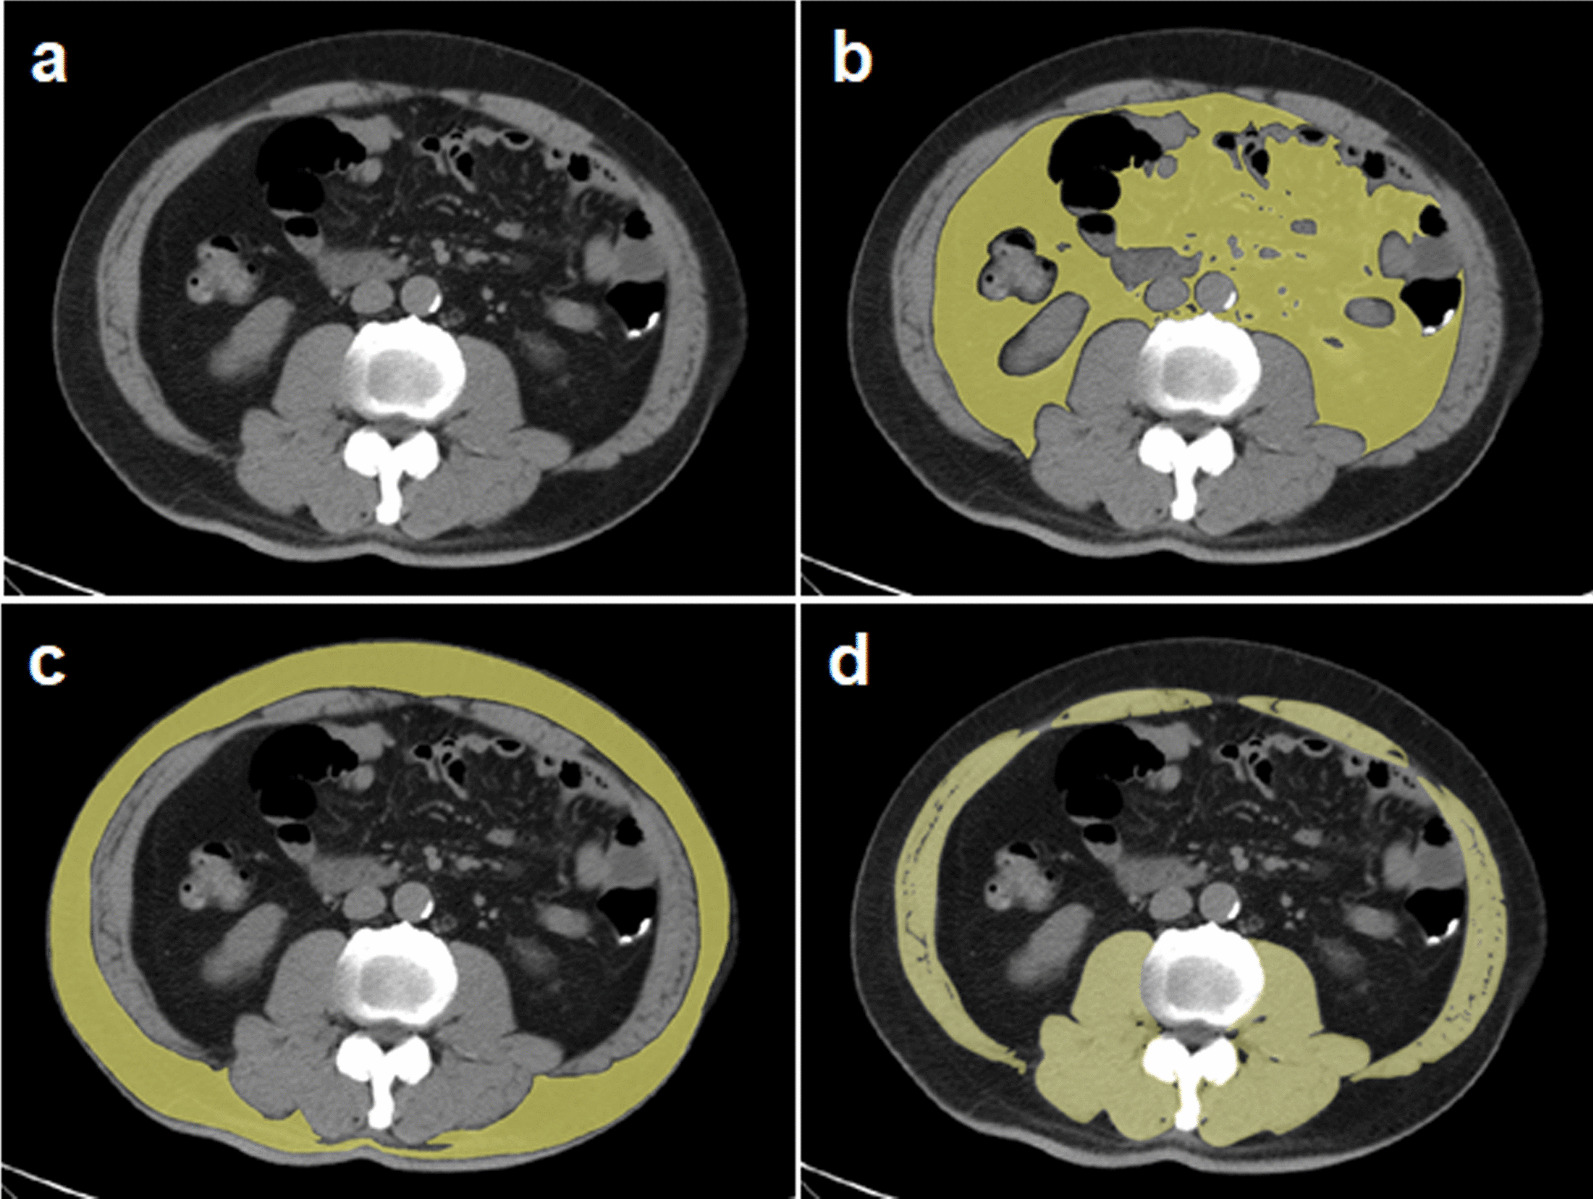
Body composition measurements using computed tomography at the level of third lumbar vertebrae. (A) Original computed tomography images for measurements. (B) Delineation of visceral fat area with a threshold of − 150 to − 50 HU. (C) Delineation of subcutaneous fat area with a threshold of − 190 to − 30 HU. (D) Delineation of total skeletal muscle area with a threshold of − 29 to + 150 HU
